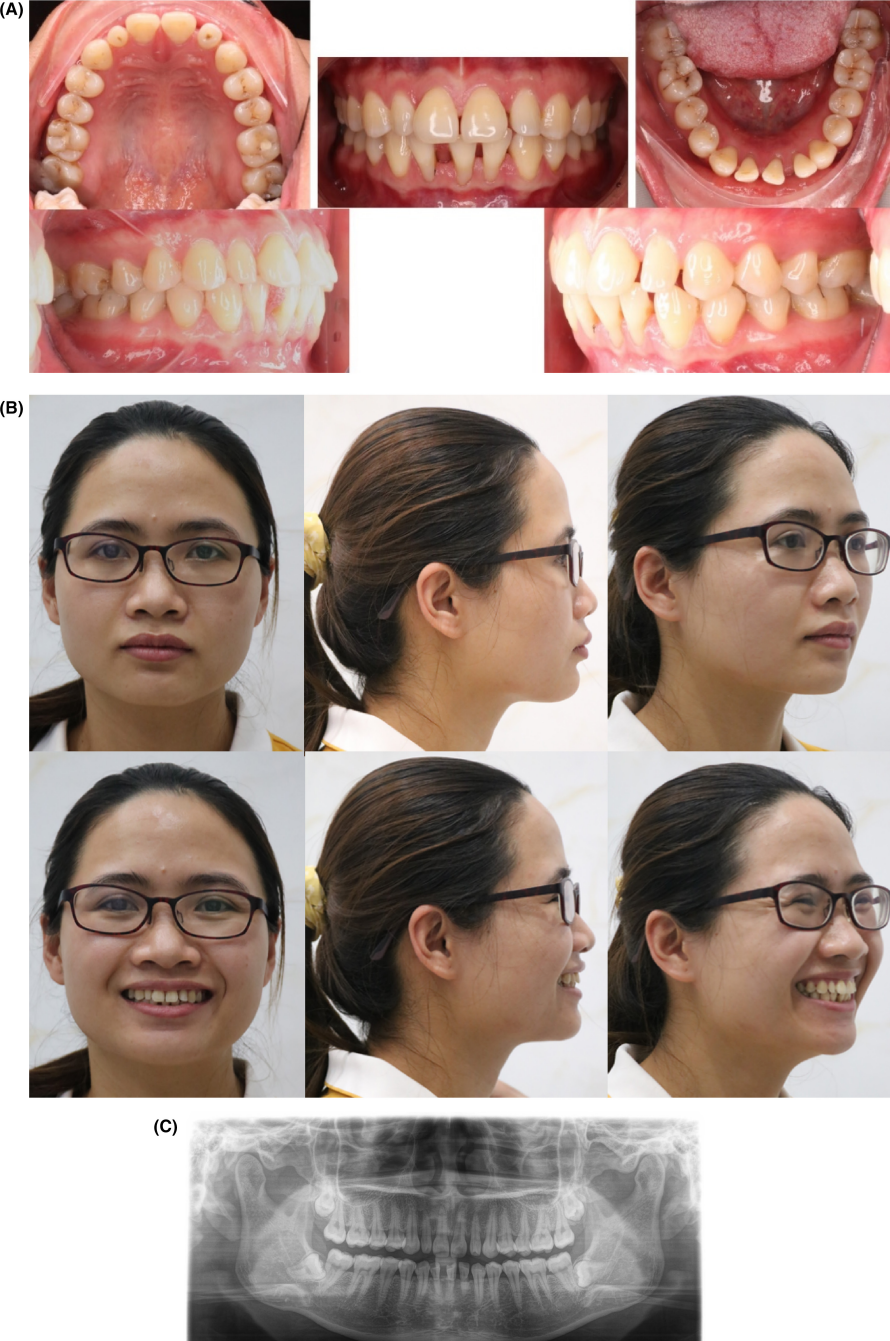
(A) Pre‐treatment Intra oral Images of the patient. (B) Pre‐treatment Extra Oral Images of the patient. (C) Pretreatment Xrays.
